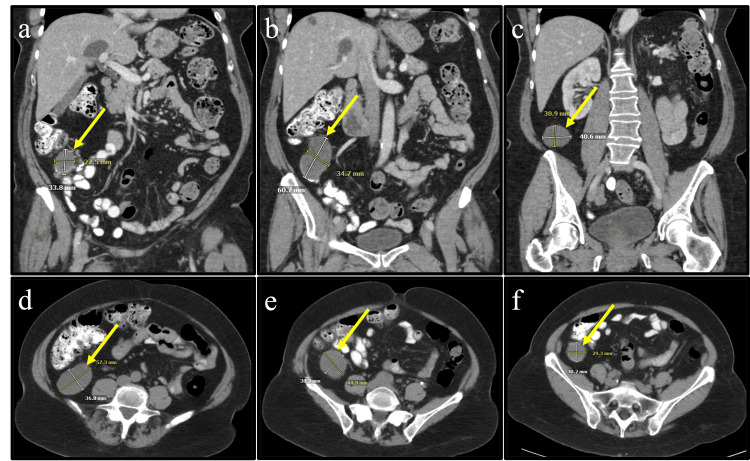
Contrast-enhanced CT imaging highlighting the proposed peritoneal duplication cyst (a) coronal view of the anterior most point of the cystic lesion; (b) coronal view of the middle portion of the cystic lesion; (c) coronal view of the posterior most point of the cystic lesion; (d) transverse view of the superior most point of the cystic lesion; (e) transverse view of the middle portion of the cystic lesion; and (f) transverse view of the inferior portion of the cystic lesion. Note the lack of continuity and absence of noticeable attachment to the appendix or cecum. An absence of contrast within the lesion lumen also suggests separation from the digestive tract. Findings suggest a peritoneal duplication cyst rather than an appendiceal mucocele.
